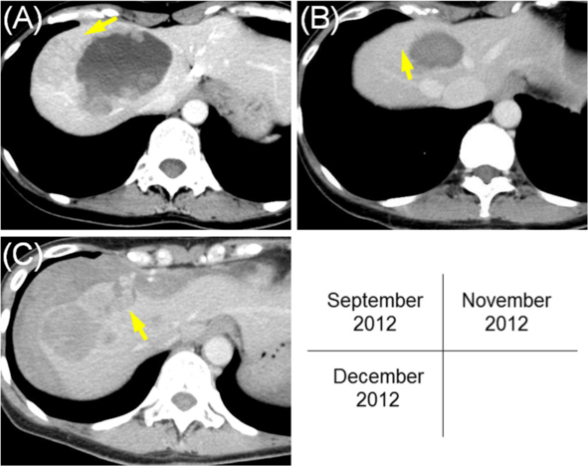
Sequential images of abdominal computed tomography (CT). These images are from September 2012 (a), November 2012 (b), and December 2012 (c). There is a metastatic tumor in the right lobe of the liver (a). Initially, the hepatic metastases considerably respond to vemurafenib treatment, and they become almost invisible (b). Later, they grow rapidly and rupture, resulting in a large amount of free fluid within the peritoneal cavity surrounding the liver (c). The *yellow arrow* demonstrates the same tumor in each image
